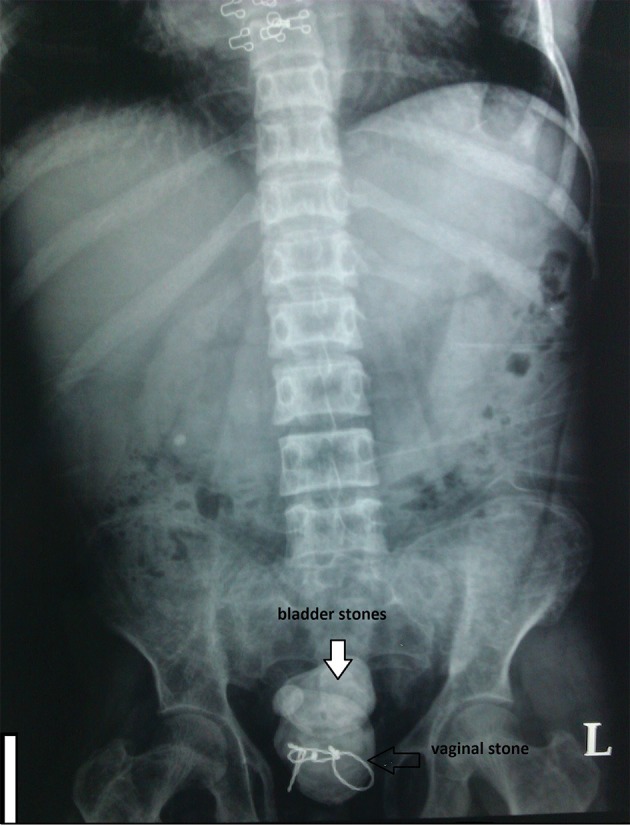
Plain Radiography of the Pati which Shows Bladder Stones and a Huge Vaginal Stone
